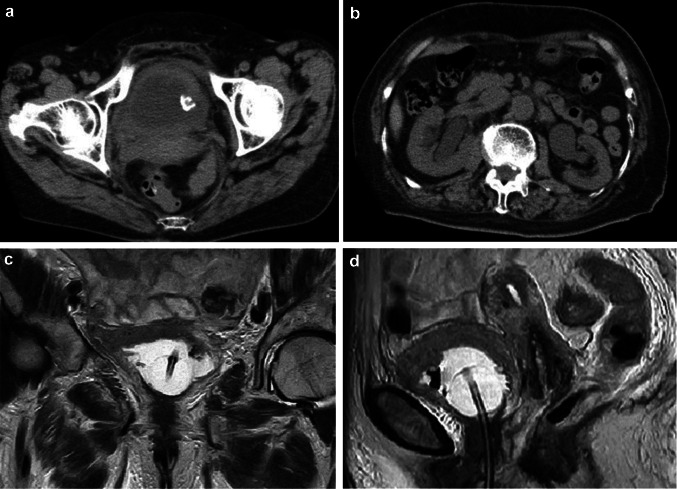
CT revealed 9 × 7 mm bladder tumor at the left top of the bladder and showing thickening of the whole bladder wall (a) and bilateral hydronephrosis (b). MRI showed the bladder trigone at coronal view (c) and relationship between bladder and uterus at sagittal view (d). These views ruled out locally advanced uterine cervical cancer
